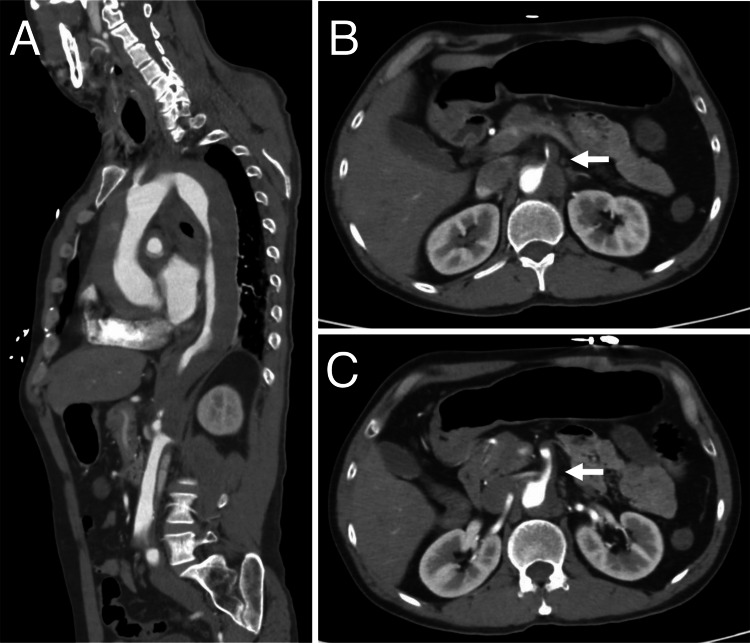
Dynamic contrast-enhanced CT revealing a Stanford type A aortic dissection. A 52-year-old man was transported to the hospital by ambulance with acute back pain as his primary complaint. The sagittal image (A) from the dynamic contrast-enhanced CT scan reveals a Stanford type A aortic dissection. The dissection extends to the celiac artery (B) and superior mesenteric artery (C), with the true lumen compressed by the false lumen.
